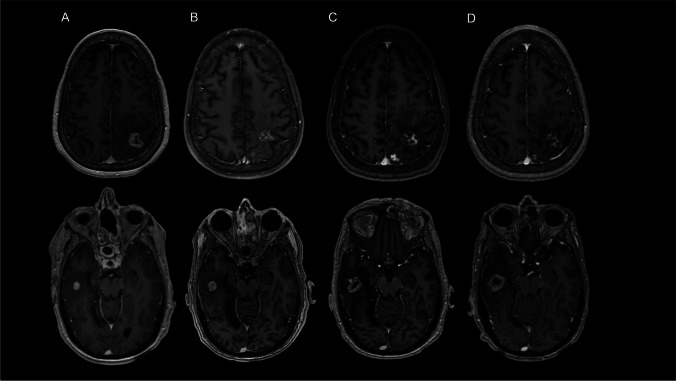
Lesions with RU for representative patients diagnosed with ARE and tumor progression. Lesions with RU on T1 post-gadolinium MRI at A) RU onset, B) 18 months after RU onset, C) 30 months after RU onset, and D) RU resolution for representative patients diagnosed with ARE (top panel) and tumor progression (bottom panel)
